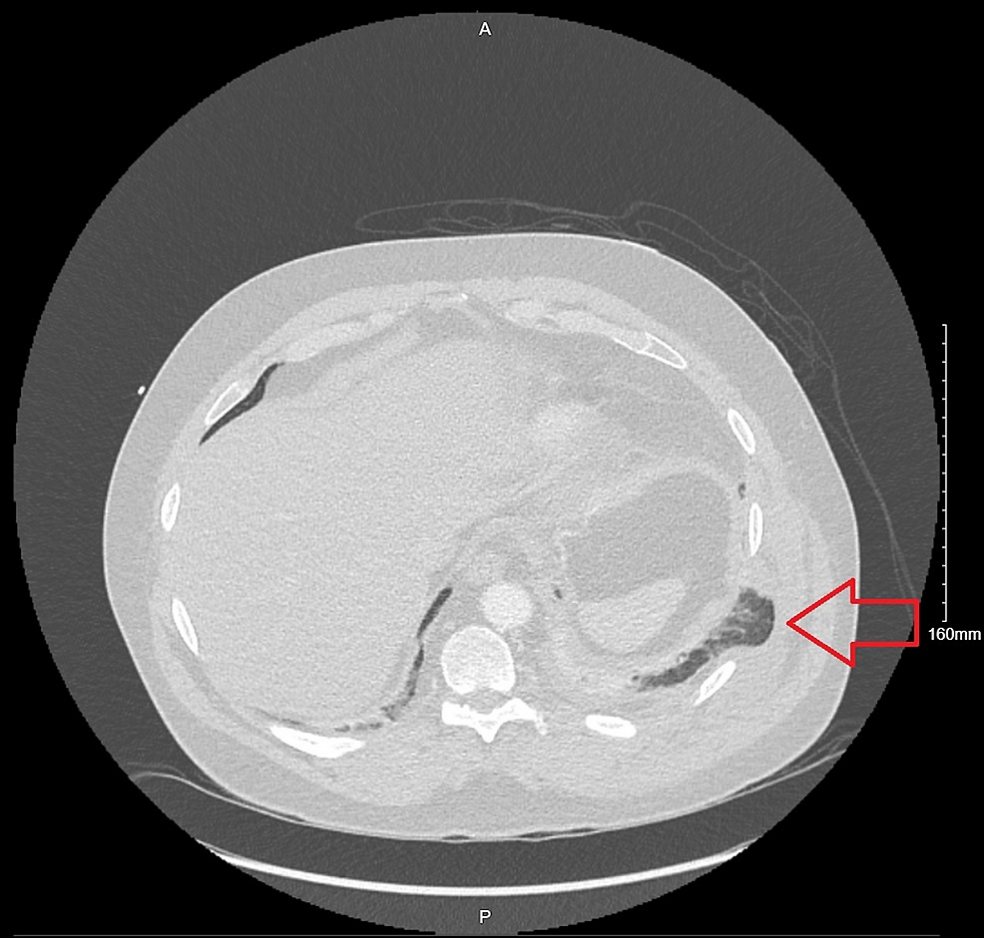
Axial view chest CT imaging showing area of intercostal widening with lung herniation on left lower thoracic region, between ribs 8 and 9 (red arrow).
